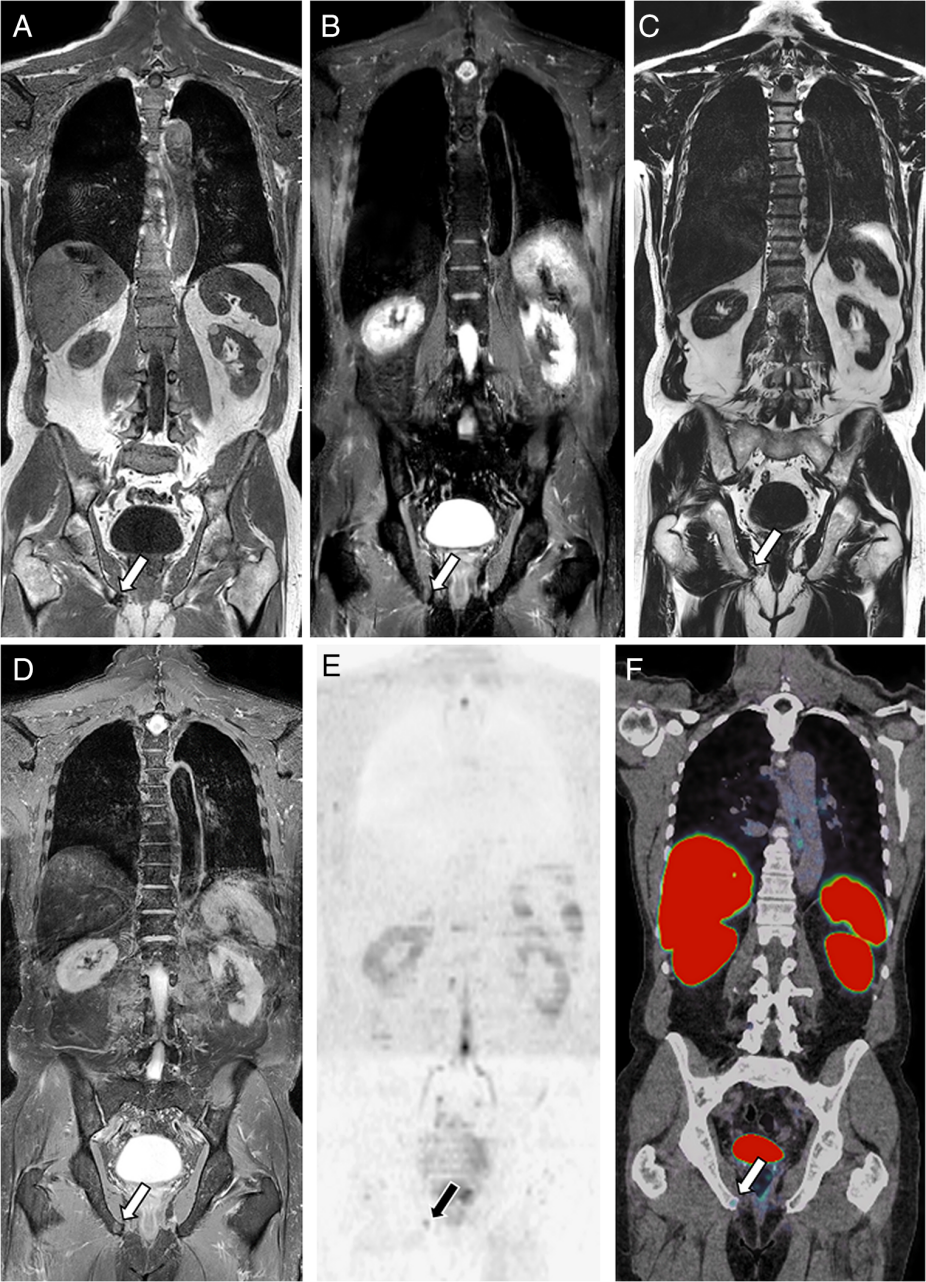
False-negative observation (detection error): WB-MRI in a 71-year-old man with newly diagnosed prostate cancer. Coronal T1 (A) and STIR (B) WB-MRI images show single centimetric bone metastasis within the right ischio-pubic ramus, with low signal intensity on T1 and high signal intensity on STIR (arrow). The lesion was missed by one observer on these T1 and STIR images. Fat (C) and water (D) reconstructions of the T2 Dixon acquisition show the same lesion with low signal intensity on the FAT image and high signal intensity on the WATER image (arrows). The reference standard, based on reading of all MR images and concurrent imaging studies, confirmed the presence of a solitary right ischio-pubic lesion. E Concurrent DWI image (*b* = 1000 s mm^−2^, inverted gray scale) confirms the presence of the lesion (arrow). F Gallium-68 PSMA fused PET/CT image confirms the presence of the lesion
